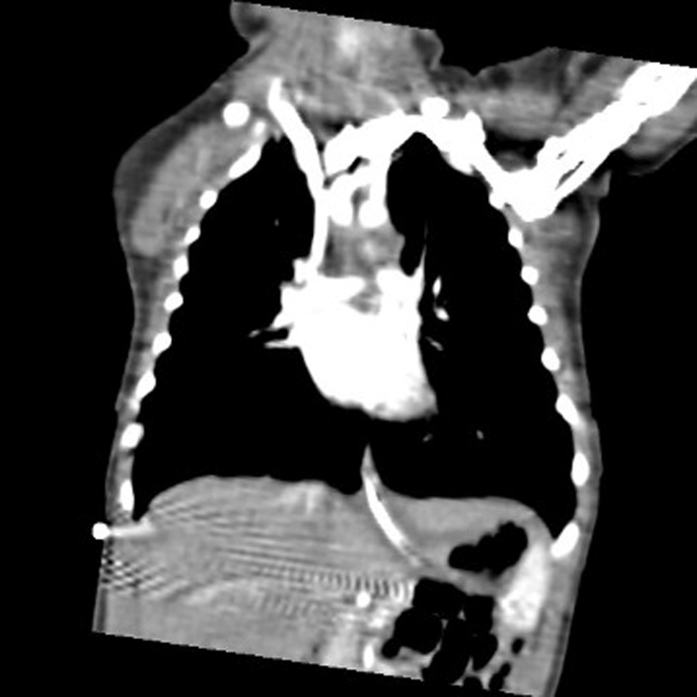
Computed Tomography Angiography of the Chest, Sagittal View, Confirming the Presence of a Double Aortic Arch
